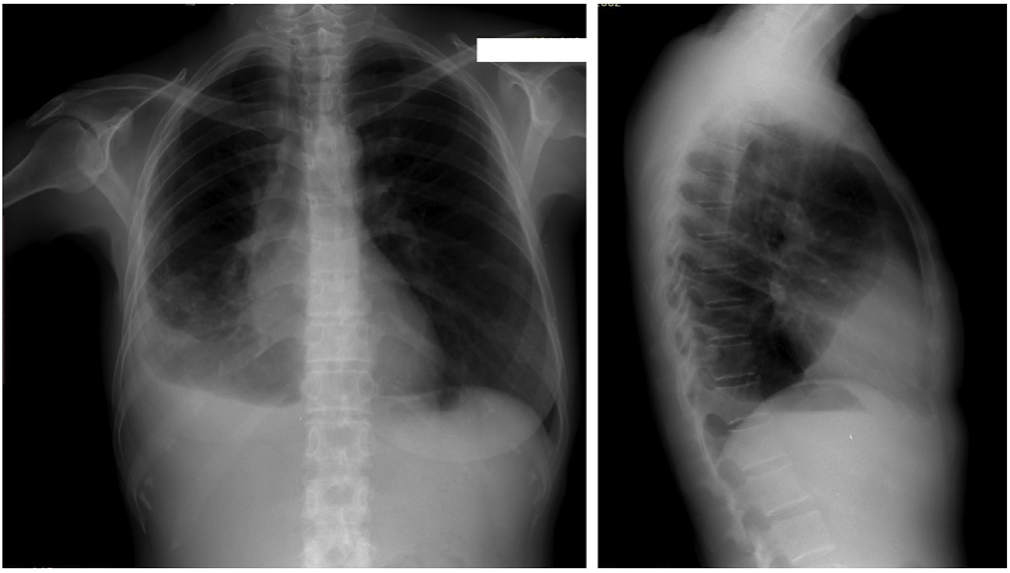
Chest X-ray at the emergency room showed a moderate right pleural effusion. In a circumstance with suspected malignancy as shown in this patient with lump and skin ulceration of the right breast with short of breath, a unilateral pleural effusion should be considered as metastatic disease. Cytology of pleural fluid confirmed the presence of malignant ductal cells.
